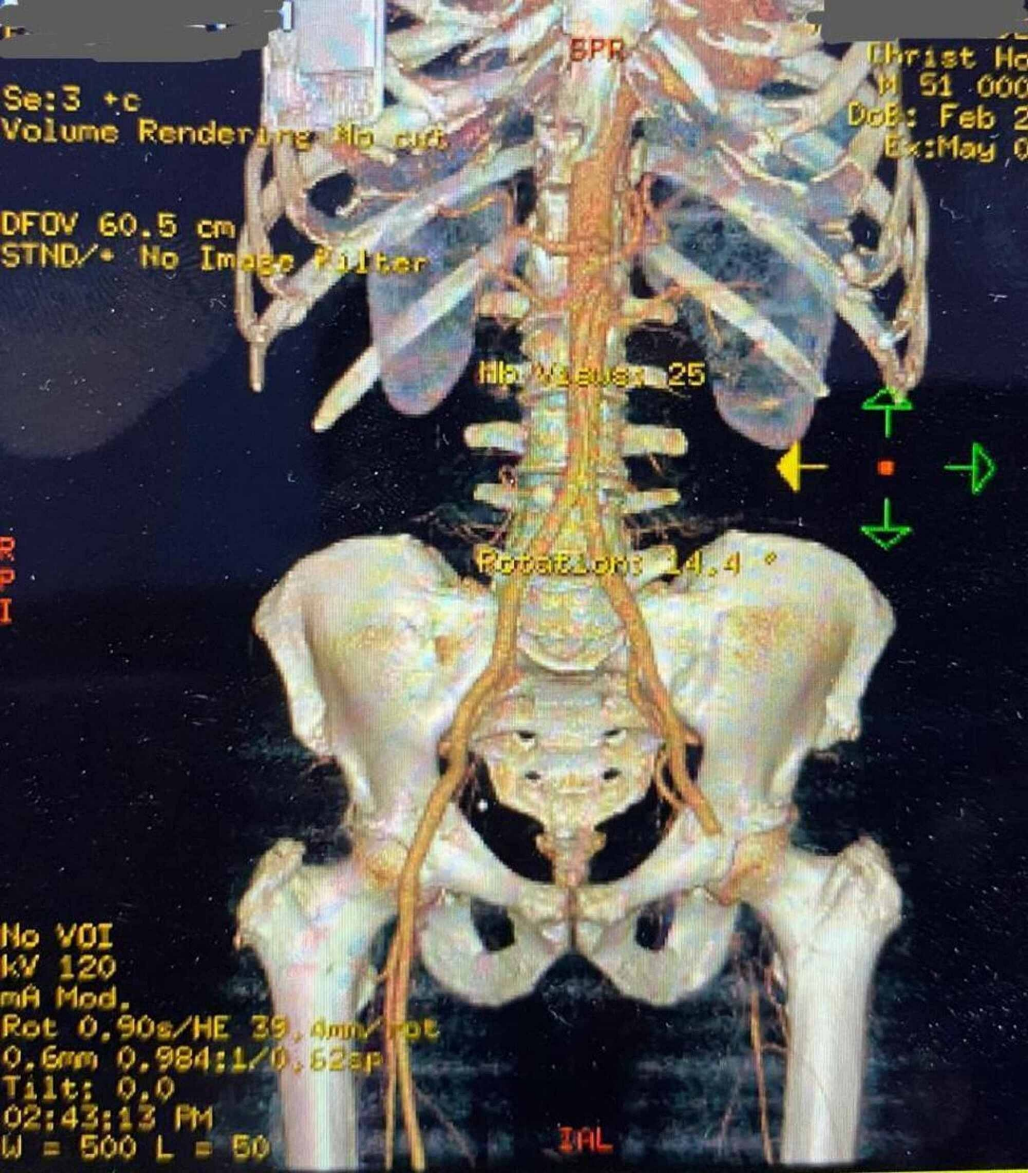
CT angiography showing an abrupt cutoff at the left common femoral artery.
